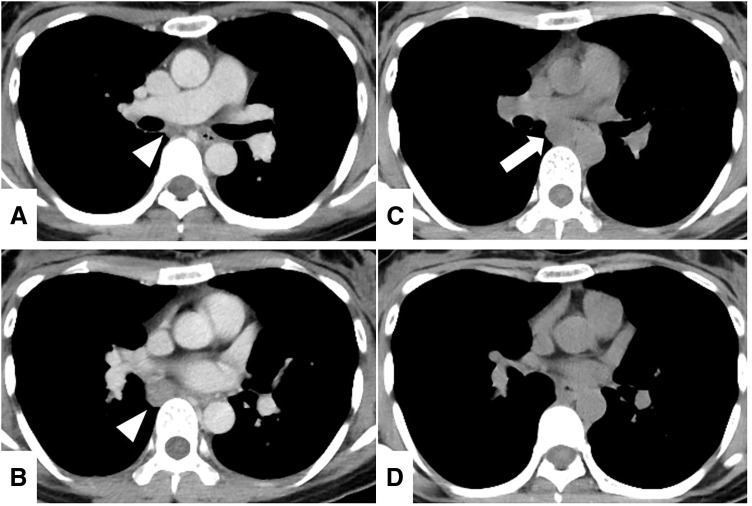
(A, B) Chest CT at the initial presentation revealed a well-circumscribed nodule along the right bronchus intermedius (arrowheads). (C, D) Chest CT 1 month later revealed that the nodule had shifted toward the midline in a cephalad direction (arrow).
